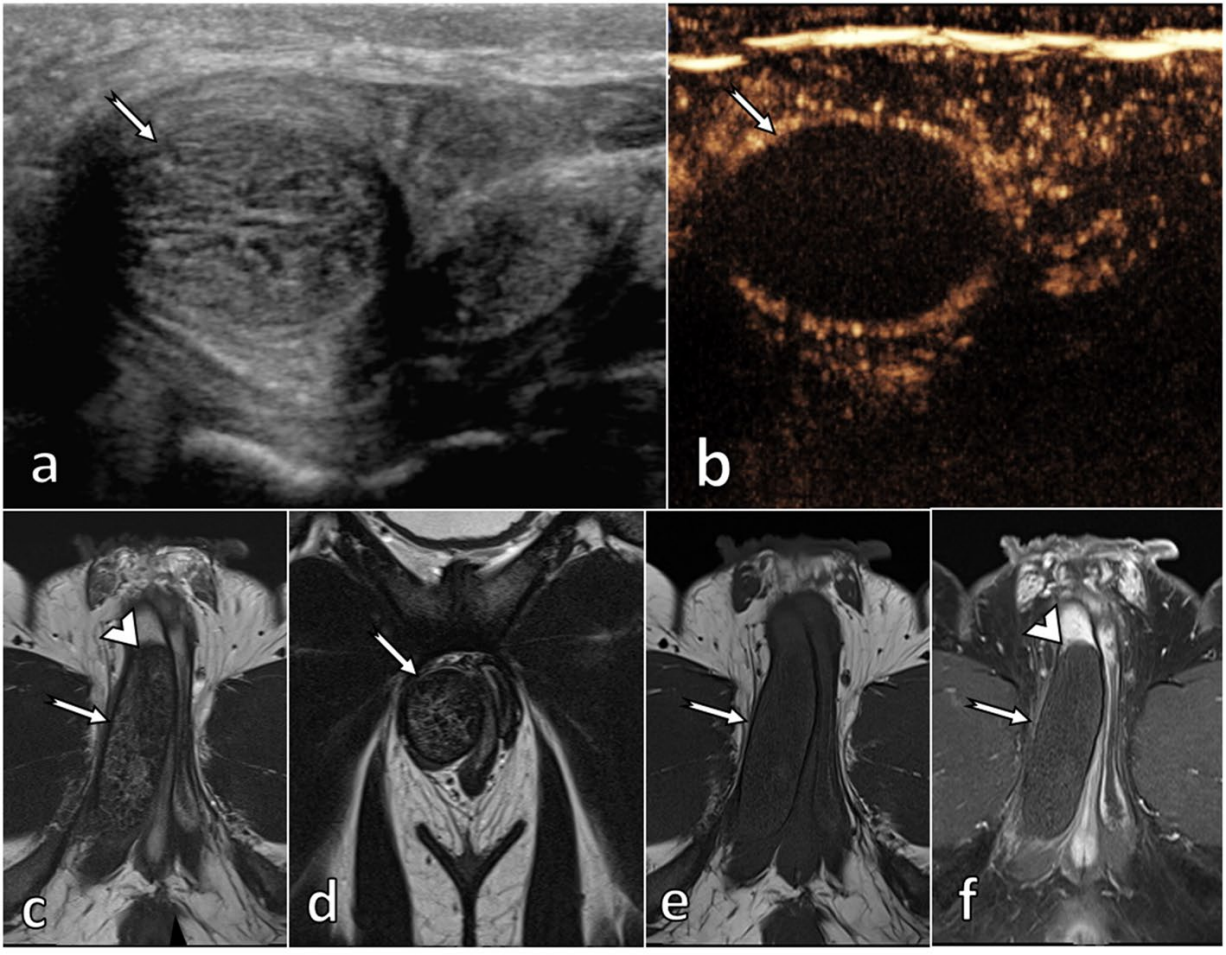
Partial segmental thrombosis of the corpus cavernosum. A 51-year-old patient, an avid mountain biker, developed a painful palpable lump in the perineum after several hours of riding his bike. US (a) shows a swollen, non-compressible right corpus cavernosum (arrow), lacking vascularisation at CEUS (b), consistent with partial segmental thrombosis of the corpus cavernosum. Diagnosis is straightforward considering history (repeated perineal microtraumas) and US features. Confirmatory MRI, however, has been done, showing a hypointense on T2WI (c, d), non-enhancing (e, f) thrombus in the crus of the right corpus cavernosum which is divided into two parts by a web (arrowhead). This is a well-known predisposing factor for partial segmental thrombosis
